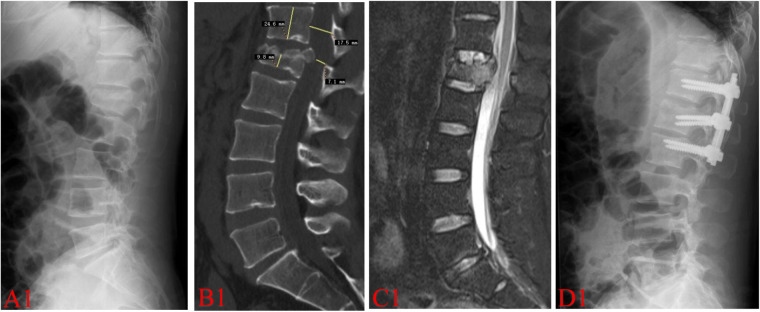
Representative case 1. A 35-year-old male presented following a motor vehicle accident. CT imaging (B1) revealed an L1 burst fracture characterized by severe comminution of both the superior and inferior endplates, vertebral height collapse greater than 50%, and spinal canal encroachment exceeding 50% due to retropulsed bone fragments; the posterior ligamentous complex (PLC) appeared intact, and no neurological deficits were observed. MRI (C1) demonstrated severe intervertebral disc injury. The patient received a TLICS score of 2 (indicating conservative treatment), whereas both NmTLICS and CN-mTLICS assigned a score of 4 (indicating an indeterminate recommendation). Ultimately, the patient underwent posterior spinal fixation from T12 to L2 (D1). In this case, TLICS failed to align with the actual treatment regimen, while NmTLICS and CN-mTLICS successfully predicted the surgical intervention. CT, Computed Tomography; MRI, Magnetic Resonance Imaging; TLICS, Thoracolumbar Injury Classification and Severity Score; NmTLICS, Novel Modified Thoracolumbar Injury Classification System; CN-mTLICS, China-modified Thoracolumbar Injury Classification System.
